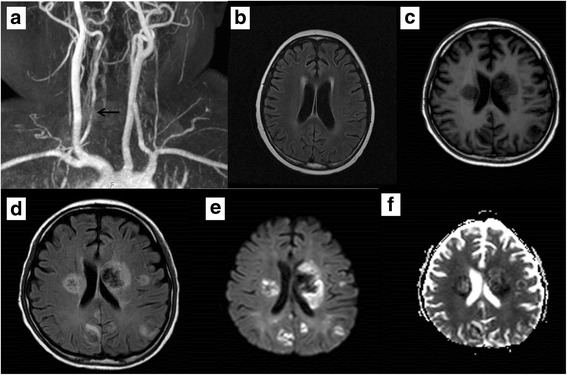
Brain magnetic resonance imaging (MRI). a) MR angiogram showed right vertebral artery narrowing (arrow) (Case 3). b) Axial T2-weighted flair MRI imaging demonstrated hyper-intense lesion on the peri-ventricular white matter (TR/TE: 9002/135.4 ms) (Case 3). c–f) Acute hemorrhagic encephaomyelitis (Case 8). Axial T2-weighted flair showed multiple hyper-intense with central hypo-intense lesions on the thalamus and white matter (T1-weighted flair: TR/TE: 2000/20 ms; T2-weighted flair: TR/TE: 8000/125 ms; ADC and DWI: TR/TE: 6000/72.89 ms)
